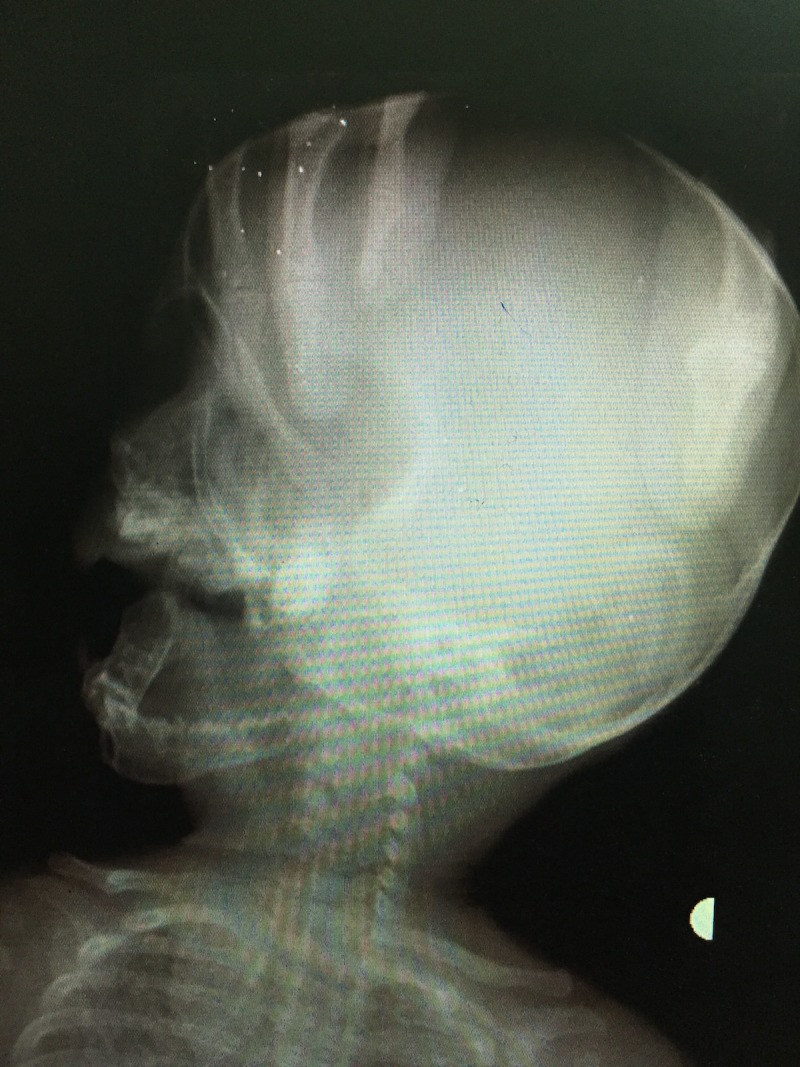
Lateral view of skull and mandible Radiograph shows normal mandible with no cortical thickening. Calvarial bones and clavicle appear normal.
